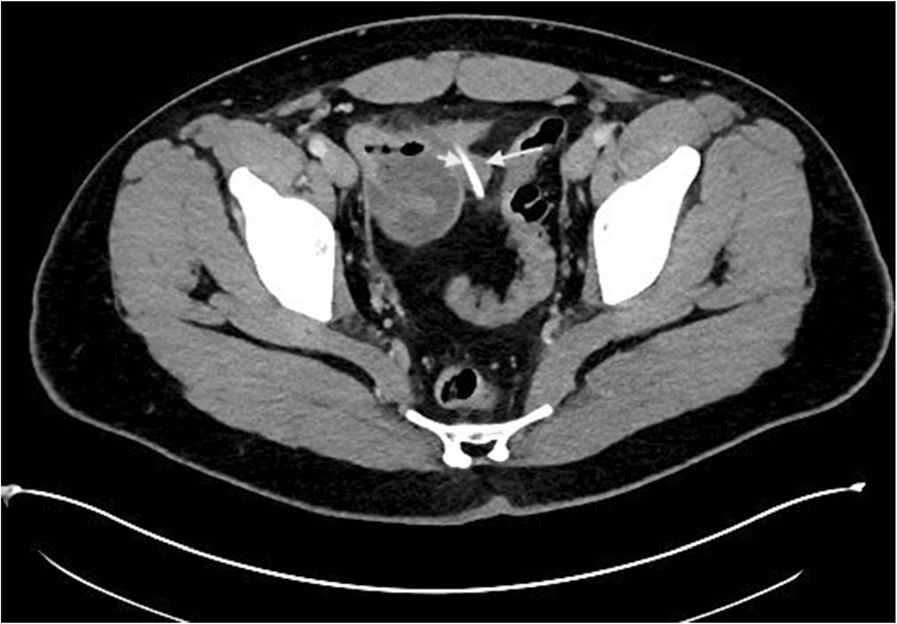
CT transverse view shows chicken bone (arrow head) inside MD (arrow)
